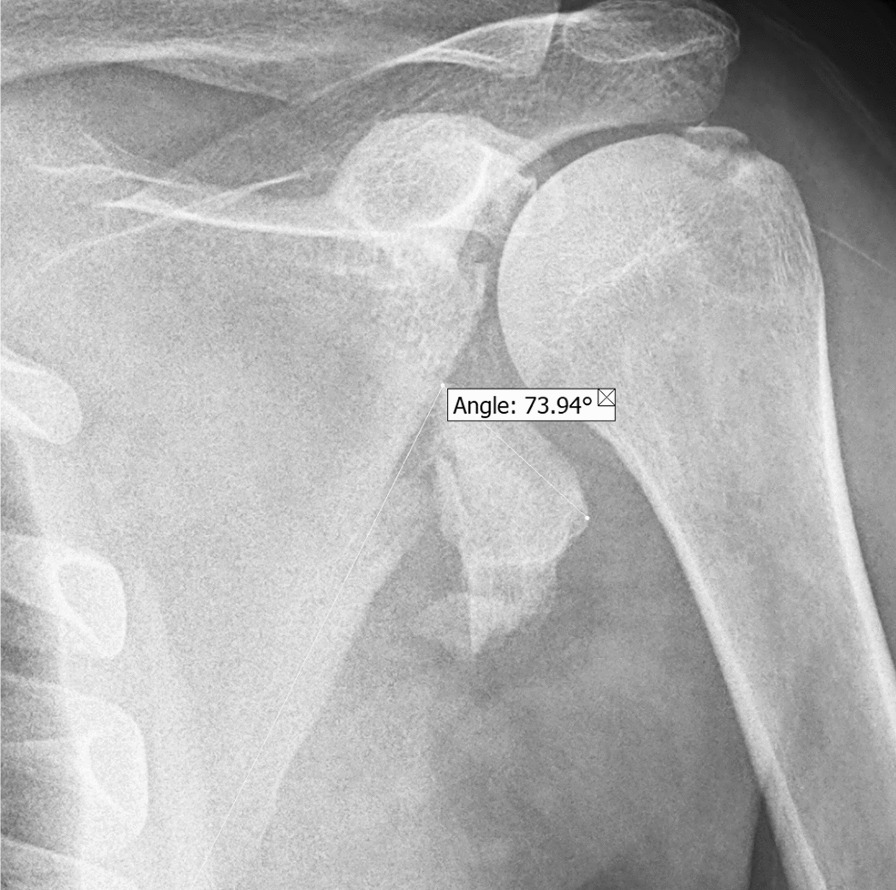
Preoperative X-ray. The humeral greater tubercle avulsion fracture and scapula anatomical neck fracture, along with an increase in the glenopolar angle, are evident in this photo
